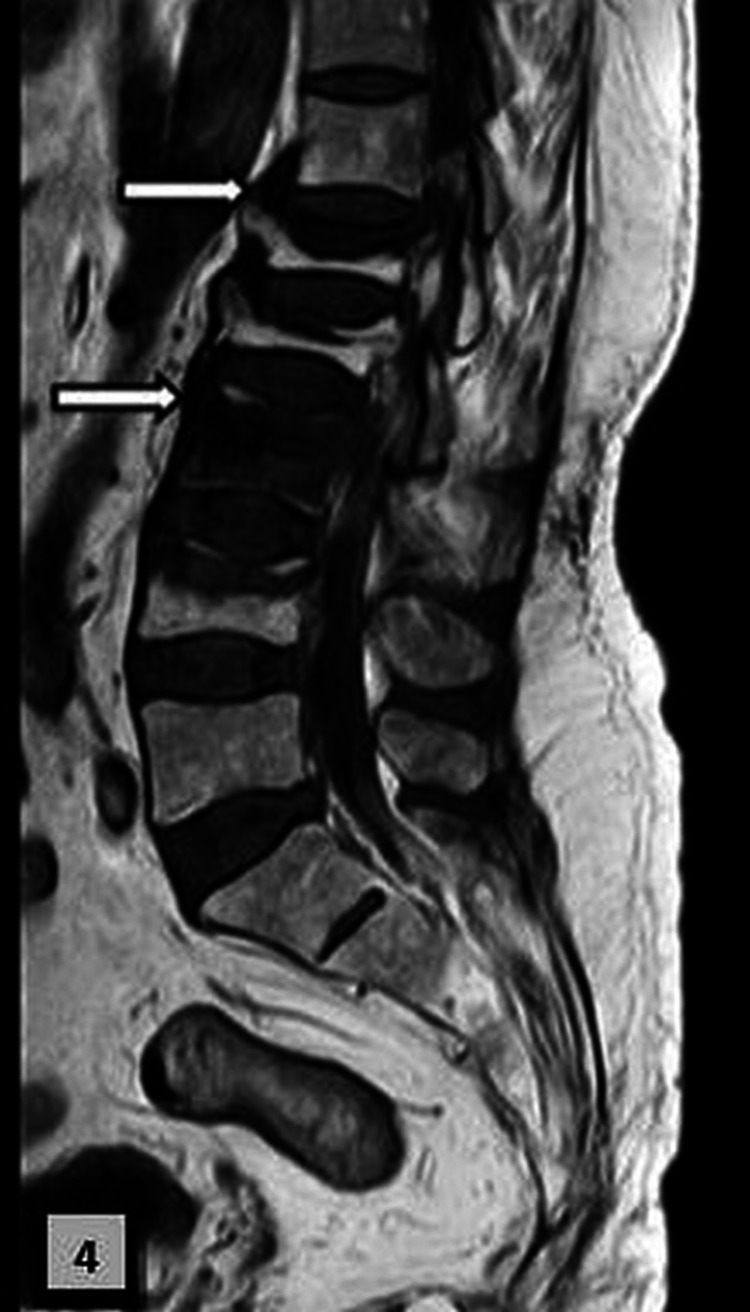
Magnetic resonance image of the lumbosacral spine (sagittal T1-weighted image) Hypointense signals below the superior endplates of L1 and L3 (arrows) are shown. L: lumbar.
